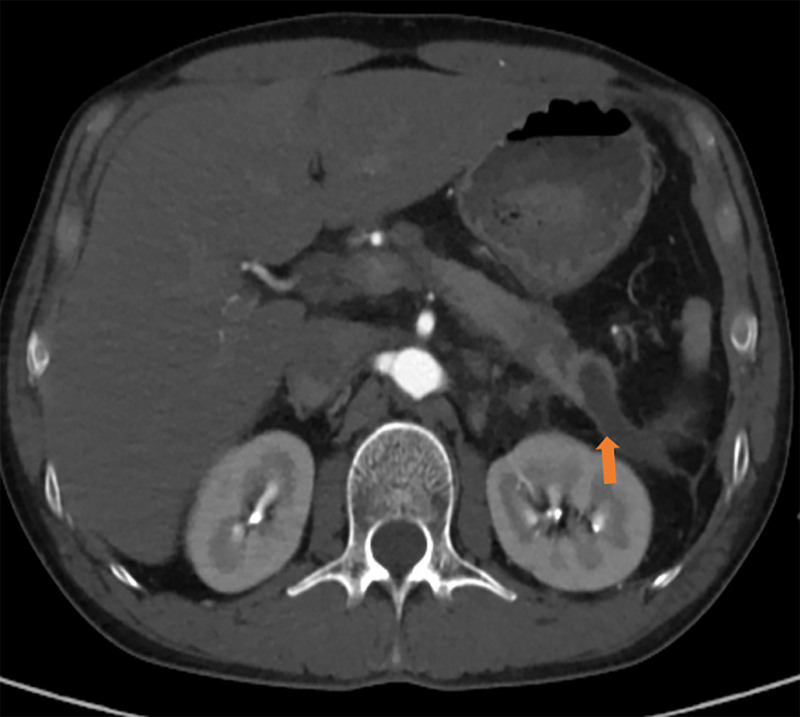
Contrast-enhanced MRI of the upper abdomen, the arrow indicates cystic lesion at the tail of the pancreas.
